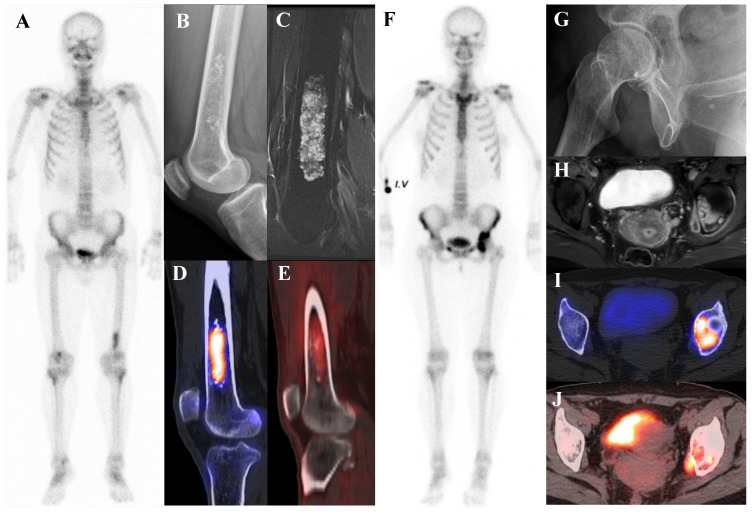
(A–E) A 54-year-old male with atypical cartilaginous tumor (ACT) of the left distal femur. (A) Bone scintigraphy shows increased uptake in the distal metaphysis. (B) Radiograph demonstrates stippled calcifications suggestive of a chondroid tumor. (C) T2-weighted fat-suppressed magnetic resonance imaging (MRI) shows a lobulated intramedullary lesion with heterogeneous signal and equivocal cortical erosion. (D) Bone single-photon emission tomography (SPECT)/computed tomography (CT) demonstrates focal uptake in the distal femur, corresponding to a calcified chondroid mass. (E) [^18^F]FDG positron emission tomography (PET)/CT shows mild, elongated uptake (SUVmax 2.96) in the lesion with characteristic rings-and-arcs calcification on CT. Surgical excision confirmed ACT. (F–J) A 49-year-old female with high-grade chondrosarcoma of the left acetabulum (F) Bone scintigraphy shows intense uptake. (G) Radiograph demonstrates a geographic radiolucent lesion. (H) T1-weighted fat-suppressed MRI reveals an infiltrative lesion with extraosseous and intra-articular extension, heterogeneous enhancement. (I) SPECT/CT reveals increased uptake in the acetabular region, corresponding to the infiltrative lesion with extraosseous extension. (J) [^18^F]FDG PET/CT demonstrates intense localized uptake in the left acetabulum with extension into the obturator internus muscle, especially pronounced at the site of extraosseous spread (SUVmax 8.4). Initial biopsy suggested grade I chondrosarcoma; however, wide excision confirmed dedifferentiated chondrosarcoma.
